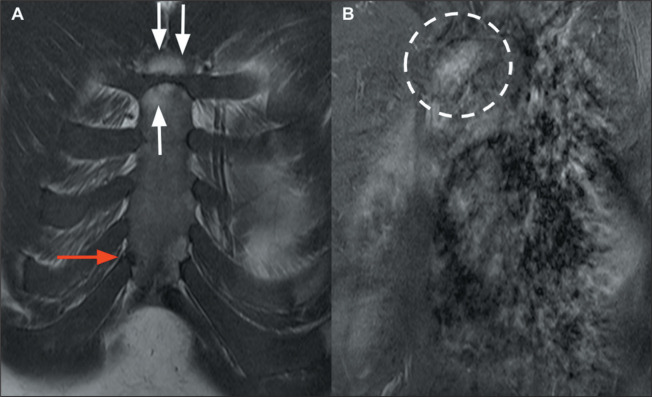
SAPHO syndrome. A: Coronal T1-weighted MRI of the sternum, showing
